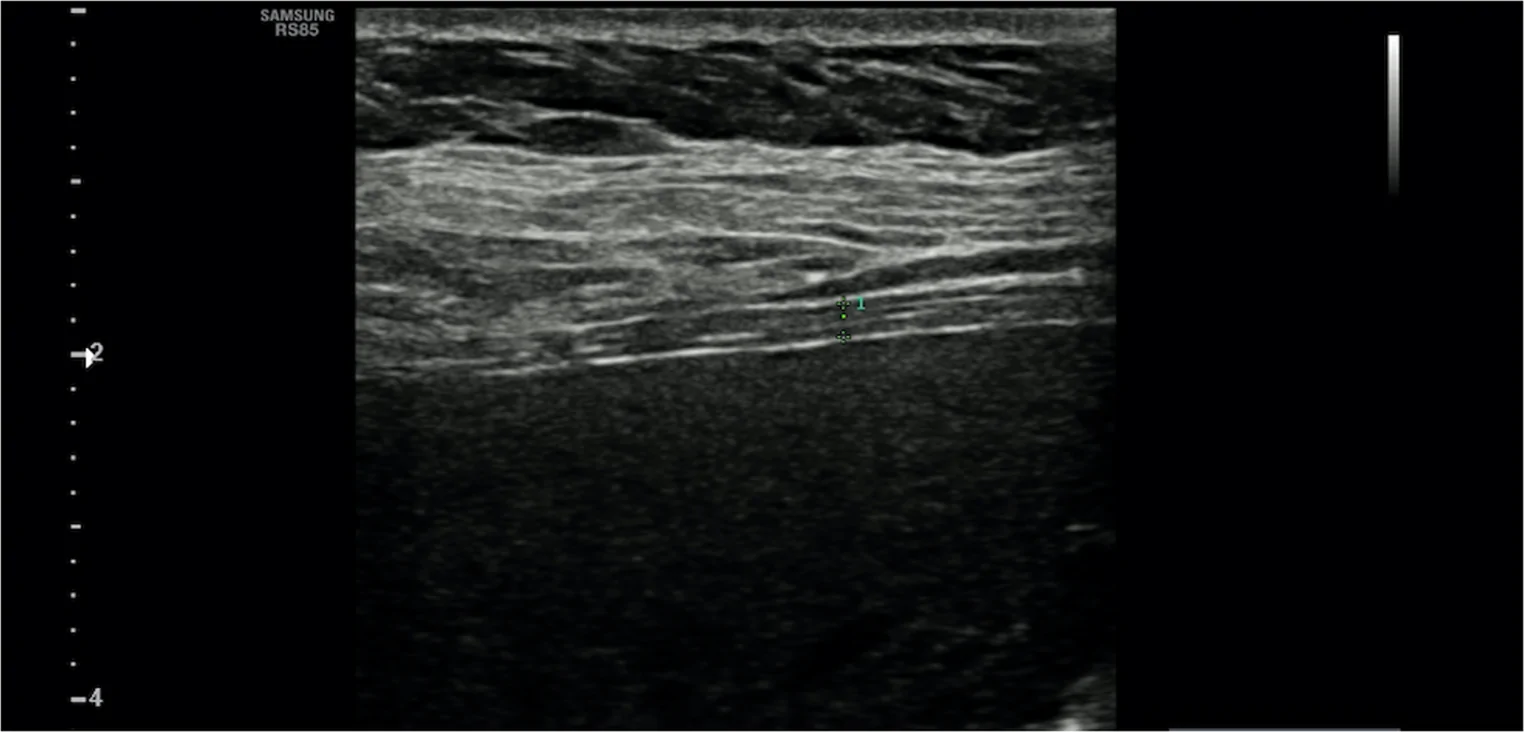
The evaluation of the thickness of the diaphragm (ultrasonography image)
